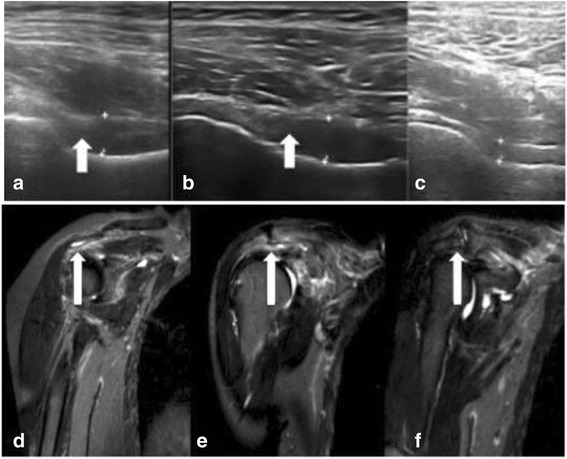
Improvements in ultrasound (US) and magnetic resonance imaging (MRI) abnormalities during tocilizumab therapy. (1) US: coxofemoral effusion at baseline (a), week 2 (b), and week 12 (c). Synovitis with synovial hypertrophy (arrows) scored 3 at baseline, 2 at week 2, and 0 at week 12. (2) MRI: subacromial bursitis at baseline (d, score = 3), week 2 (e, score = 2), and week 12 (f, score = 0)
